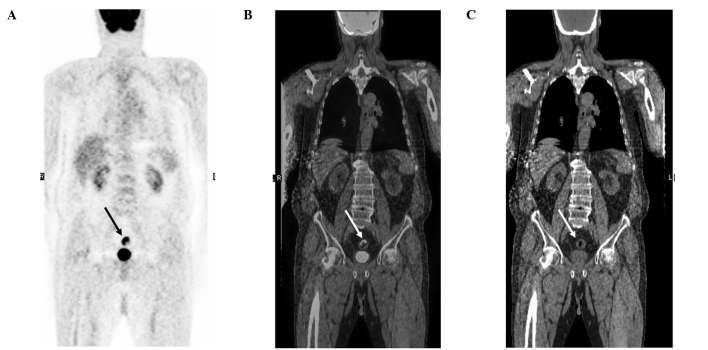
A 79-year-old male was referred for the assessment of a pulmonary nodule observed on CT. (A) Coronal FDG-PET, (B) PET/CT and (C) CT slices demonstrate intense FDG uptake in the proximal region of the colon (arrows). On colonoscopy, a large ulcer was found in 15 cm into the colon. The final diagnosis was of an adenocarcinoma of the colon. FDG PET/CT, fluorodeoxyglucose positron emission tomography/computed tomography.
